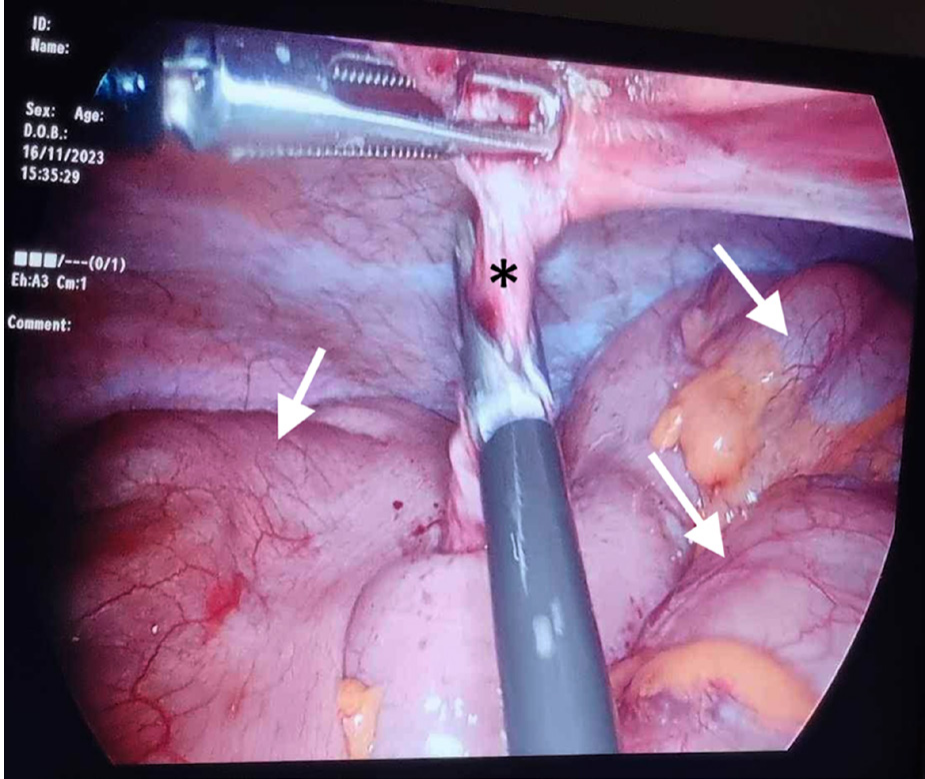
Peroperative celioscopic image showing the intestines (arrows) twisted around a cord (star), corresponding to the fibrous band seen on CT.
